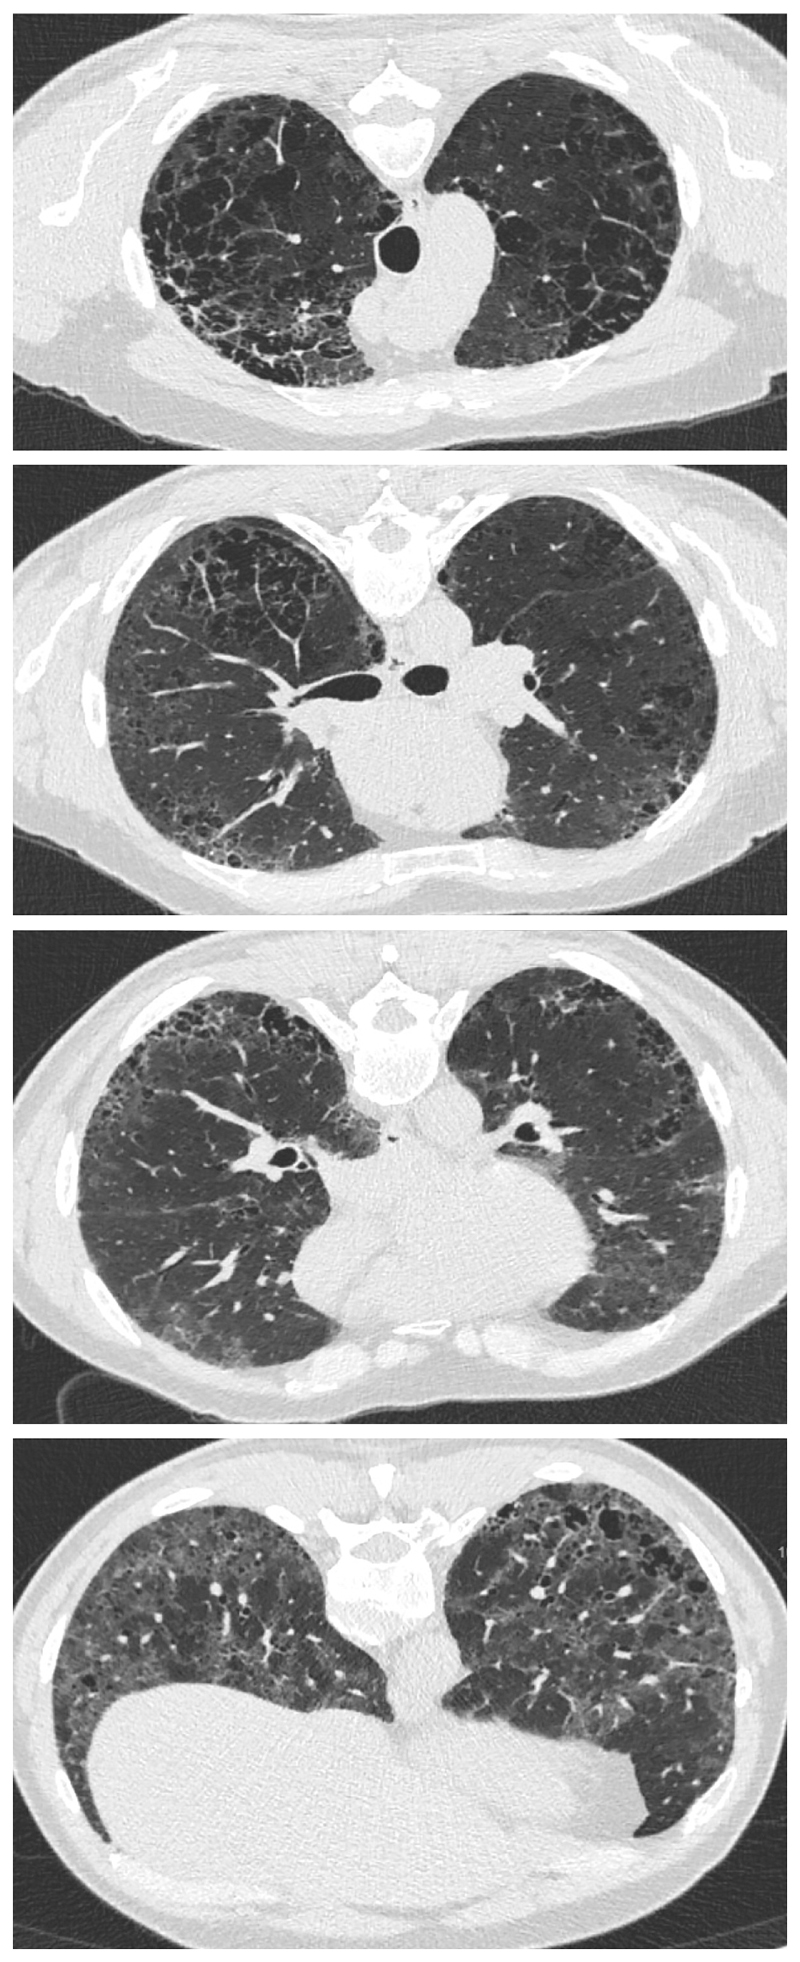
Chest HRCT showing emphysema admixed with desquamative interstitial pneumonia confirmed by lung biopsy (combined pulmonary fibrosis and emphysema, admixed pattern at HRCT). Areas of low attenuation are admixed with ground glass opacities (high attenuation) and thickening of peri-emphysematous areas.
